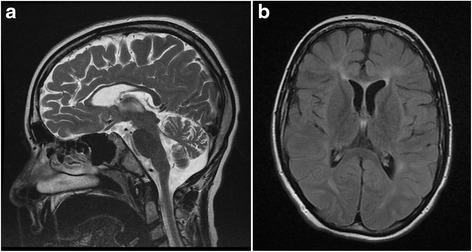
Magnetic resonance imaging scan from patient with late-infantile-onset Niemann–Pick disease type C. The magnetic resonance imaging scan shows: a vermian atrophy on T2-weighted sequence, May 2012 and; b diffuse white matter hyperintensities on fluid-attenuated inversion recovery sequence (FLAIR), May 2012
